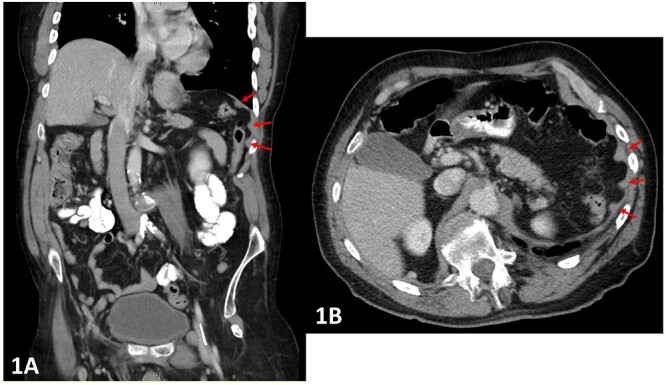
(A and B) Pre-operative CT abdomen (A—coronal, B—transverse planes) with multiple peritoneal nodules indicated by the arrows.
